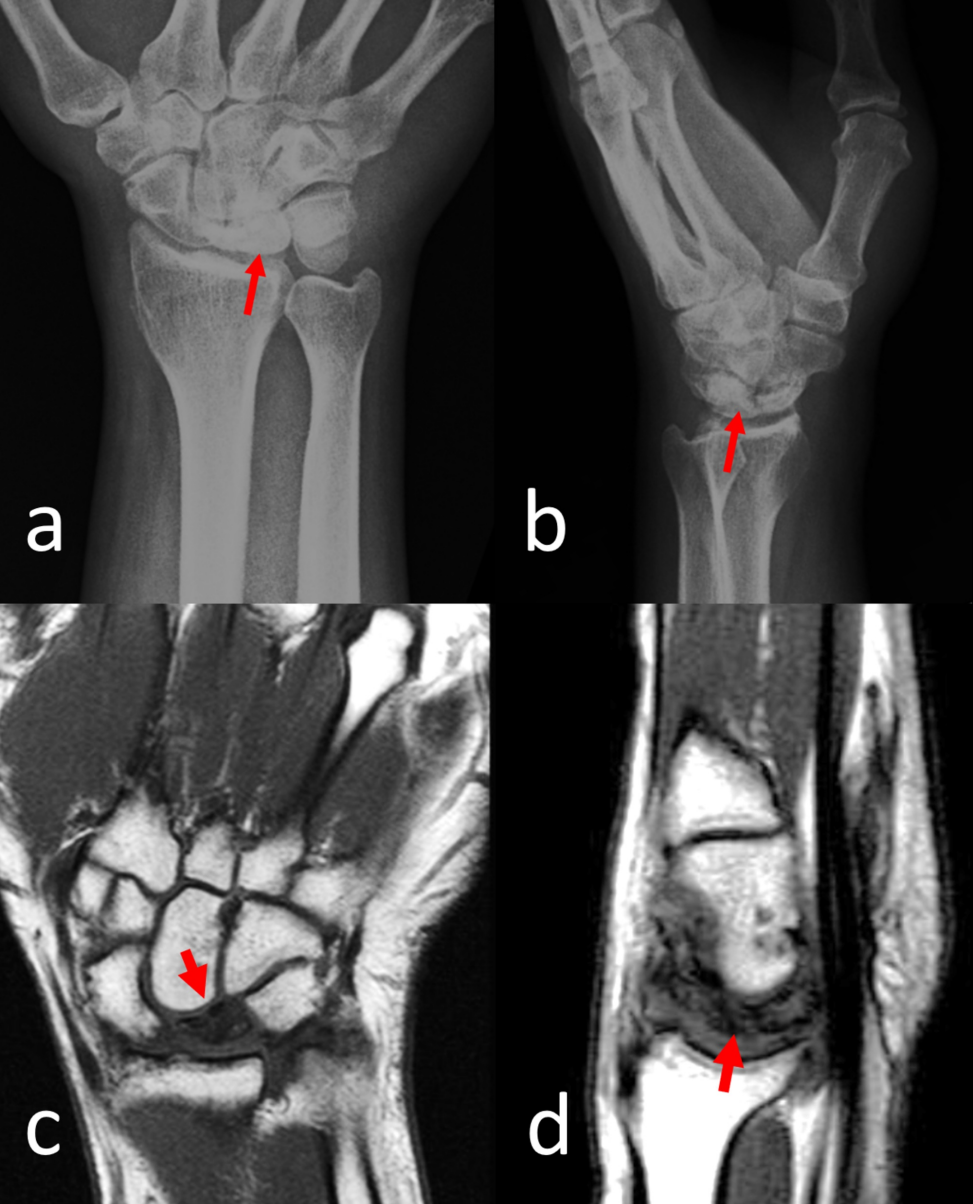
Preoperative Imaging of Case 4 A) anteroposterior and B) lateral wrist radiographs showing Stage IIIB Kienböck’s disease. The red arrows show the dense sclerotic lunate in direct radiographs. C) coronal and D) sagittal T1-weighted magnetic resonance imaging of the patient showed avascular necrosis of the lunate bone. Note the low signal intensity of the lunate demonstrating avascular necrosis (red arrows).
